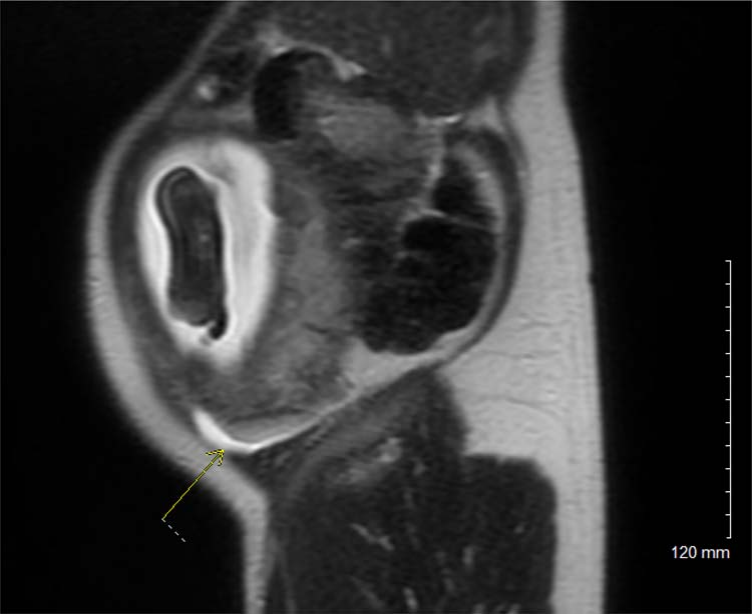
MRI pelvis, sagittal view.
